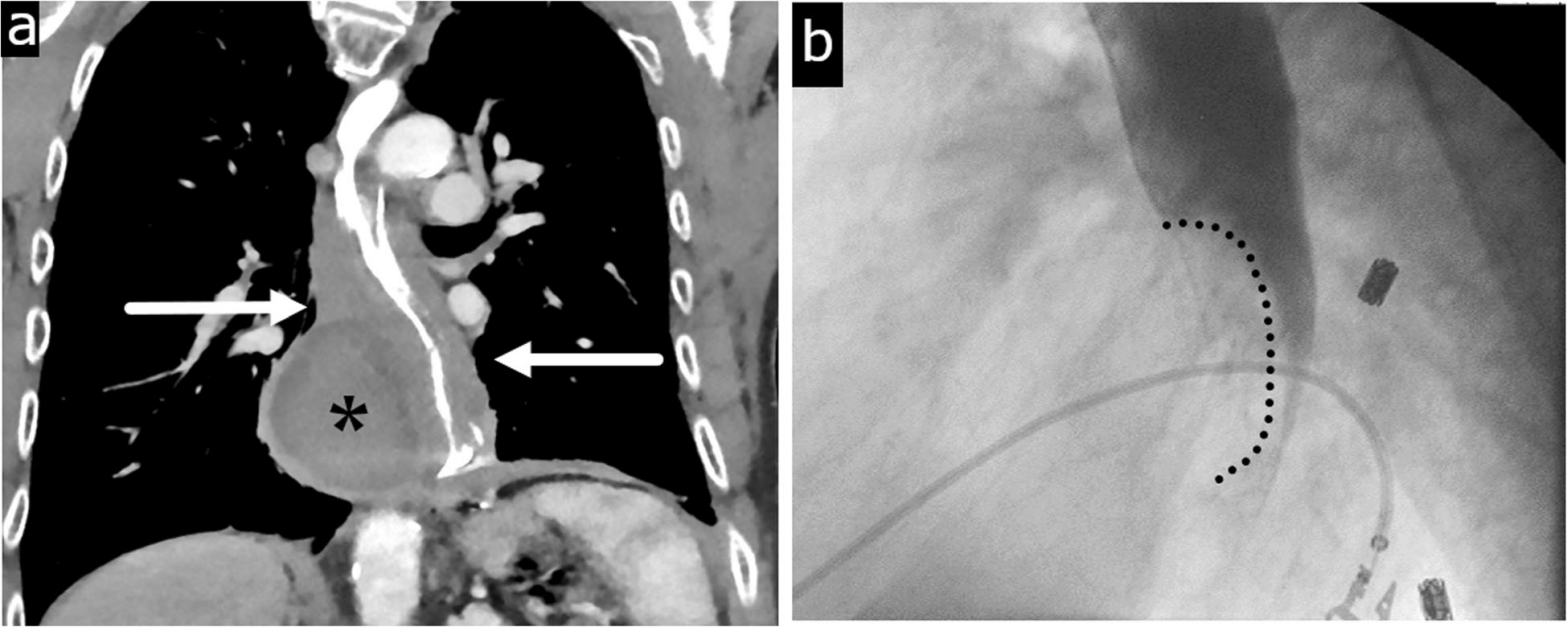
Intramural hematoma. Sixty-one-year-old male with severe chest pain and dysphagia status post recent radiofrequency ablation for Barrett’s esophagus. a Coronal CT image shows a smoothly demarcated eccentric homogeneous intramural hematoma (asterisk) in the right wall of diffusely thick-walled esophagus (arrows). b Contrast esophagography demonstrates smoothly marginated mass effect (dotted line) on the lower esophagus by the intramural hematoma
